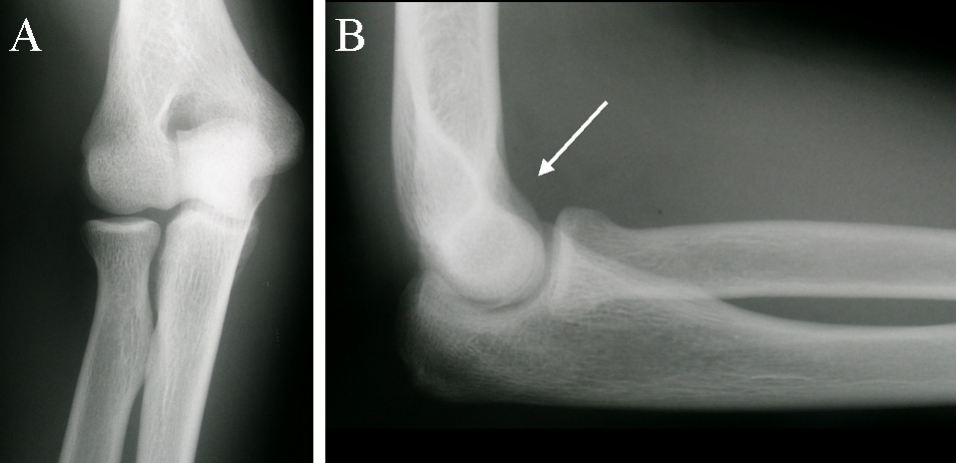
Plain radiographs of the right elbow. A. AP view, demonstrating no changes. B. Lateral view demonstrating a joint effusion (arrowed).
